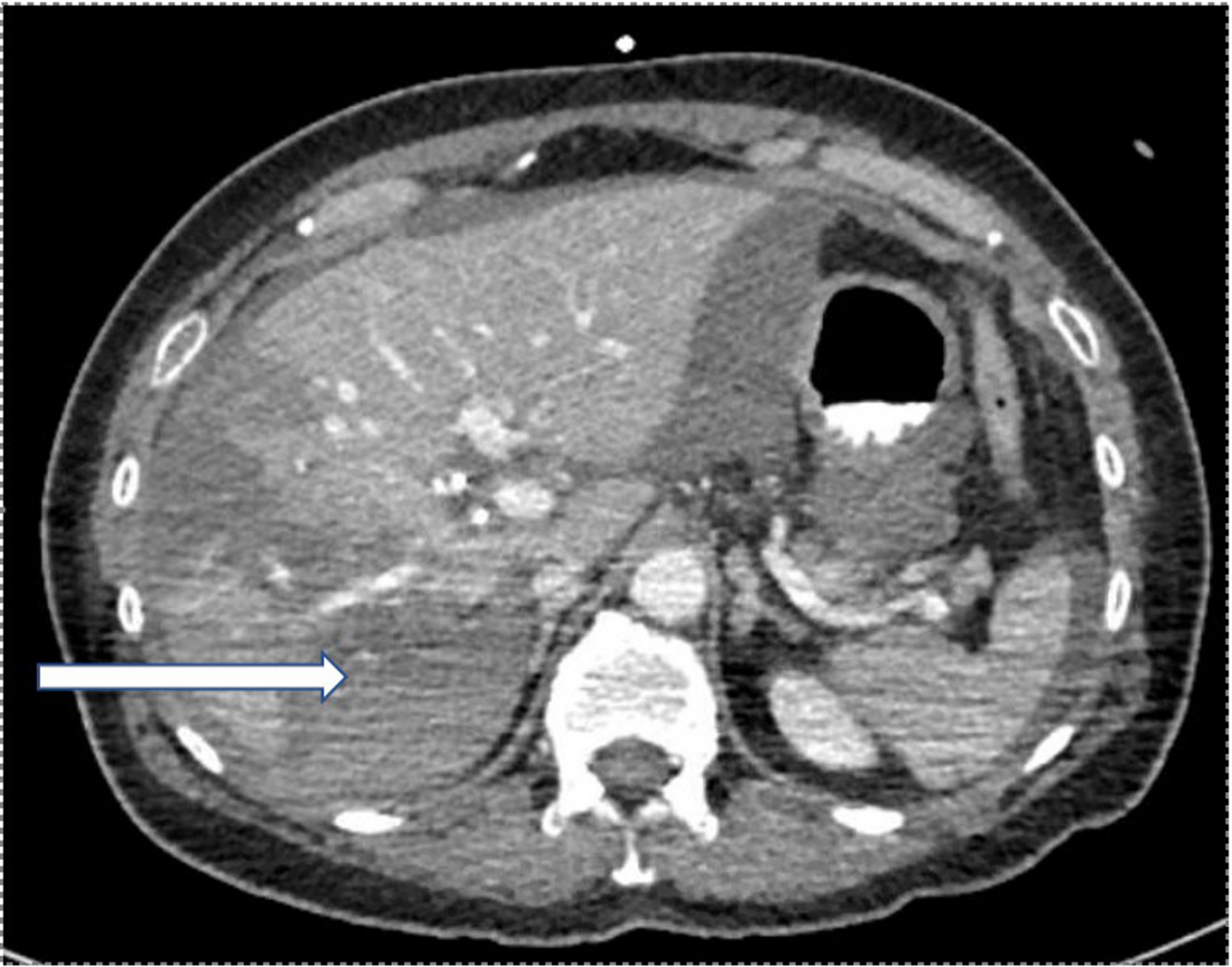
Axial contrast‐enhanced computed tomography showing perihepatic hematoma(arrow).
